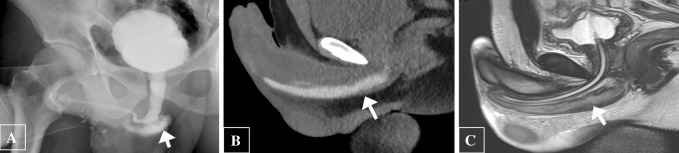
Urethral and corpus spongiosum injury in a 21-year-old gentleman with penile swelling and hematuria post intercourse. a Retrograde urethrogram depicts a urethral laceration with contrast leak into the corpus spongiosum (arrow). b CT after urethrogram shows the persistence of contrast in corpus spongiosum (arrow). c Sagittal T2-weighted MRI confirms edema and blood products within the corpus spongiosum (arrow)
